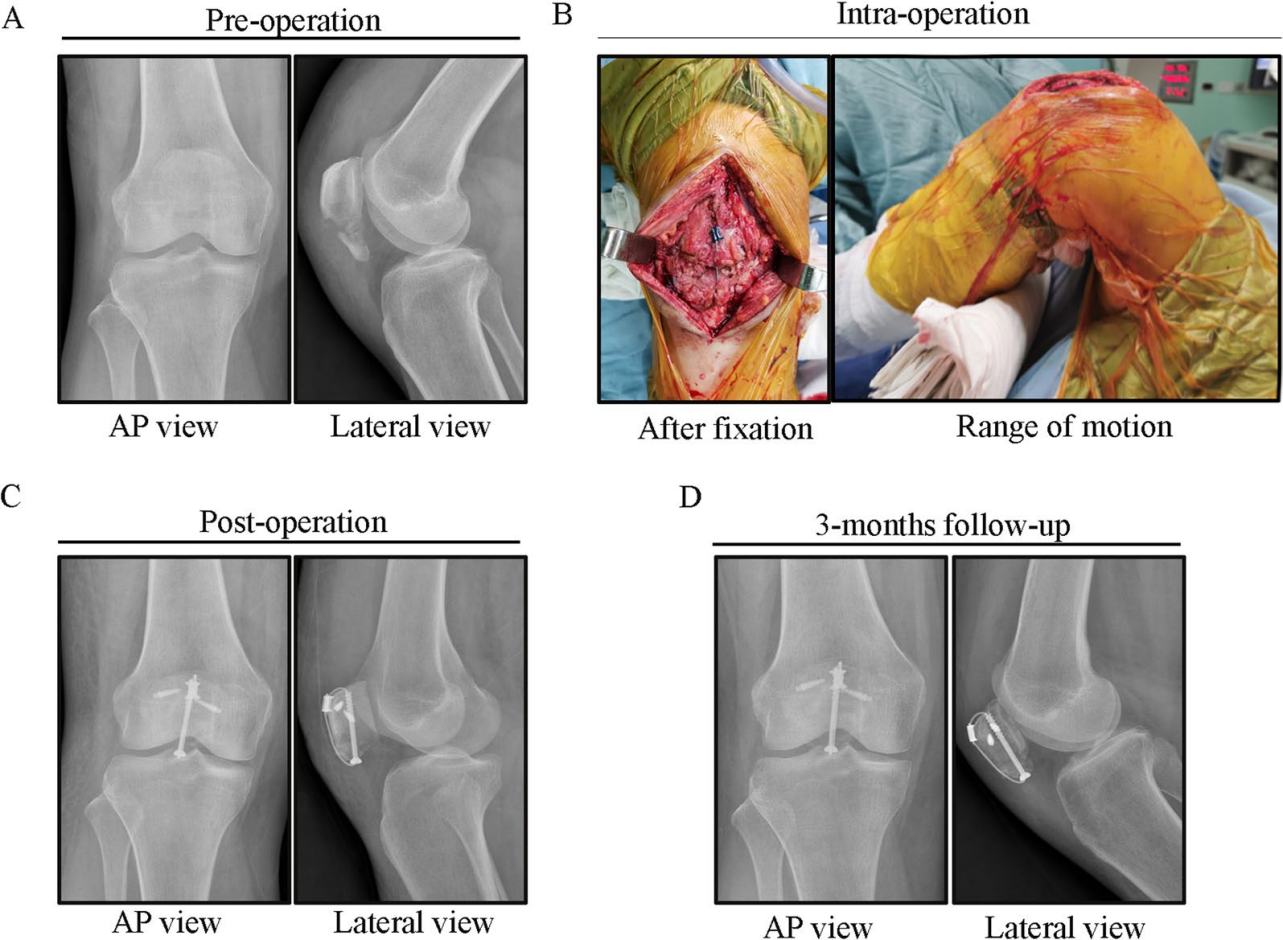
A typical case of an SVW-BSAG fixation IPFP patient. A This was a 47-year-old male patient with IPFP. B The patient underwent reconstruction of the IPFP through SVW-BSAG fixation. C Postoperative radiograph showed that the fracture was fixed in a good position. D After a 3-month follow-up, the patellar fracture healed well, and the injured knee had full range of motion
